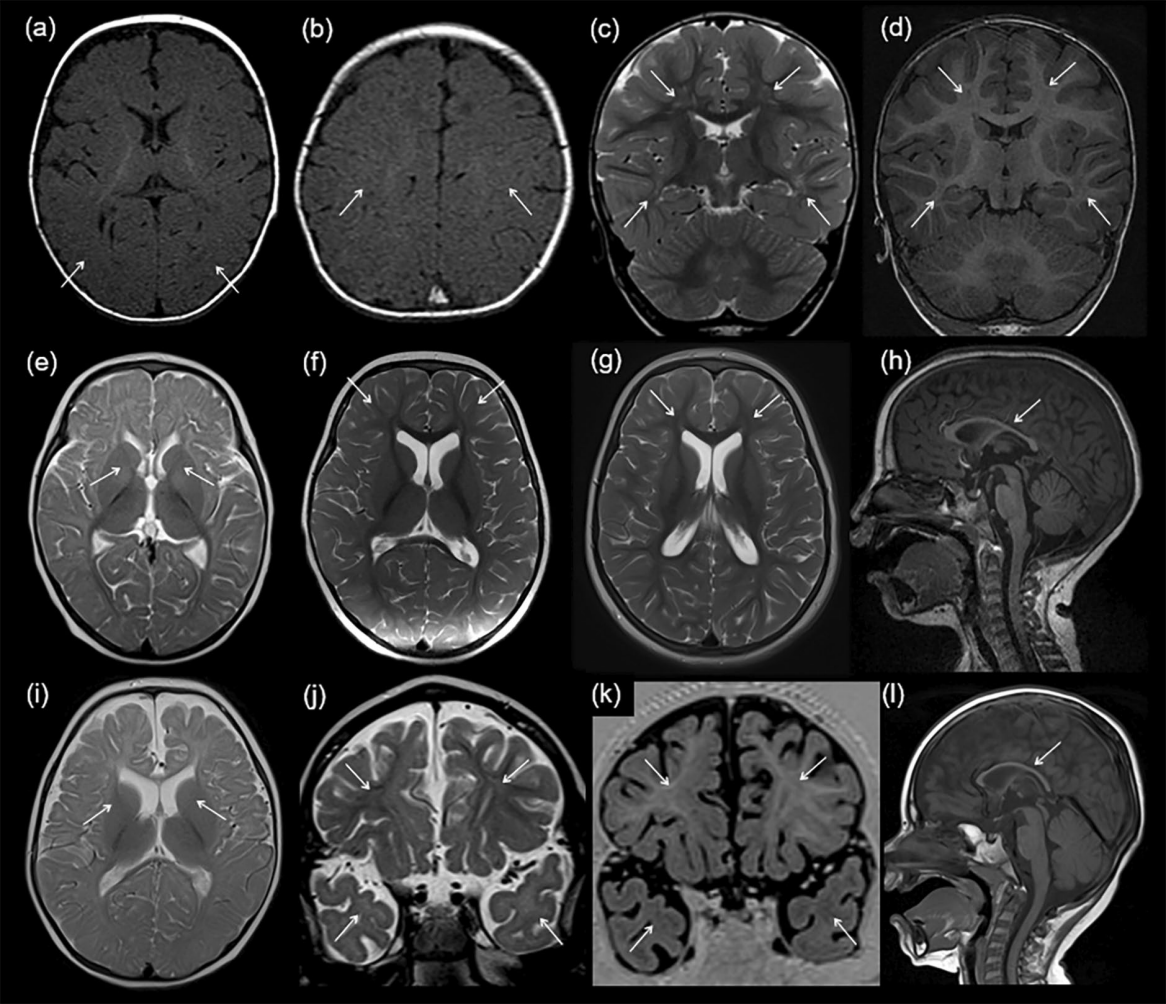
Mild hypomyelination of the diseases (early infantile epileptic encephalopathy 44 [DEE44], neurodegeneration with brain iron accumulation 5 [NBIA5], and X‐linked intellectual disability‐1 [MRX1]) previously not associated with hypomyelination. Axial T1‐weighted images of a 5‐month‐old patient with DEE44 do not present myelination of deep subcortical occipital white matter (a, arrows) or mid‐frontal white matter (b, arrows) as expected at this age. Follow‐up MRI of this patient with DEE44 at the age of 4 years shows slightly patchy frontal and temporal hypomyelination on coronal T2‐weighted (c, arrows) and T1‐weighted (d, arrows) images. Axial T2‐weighted image of a patient with NBIA5 at the age of 10 months does not show myelination of the anterior limbs of the internal capsule (e, arrows). At 22 months of age, maturation has progressed but there is still slight frontal hypomyelination on axial T2‐weighted image (f, arrows). At 3 years 8 months of age this T2‐weighted image of this patient with NBIA5 (g and h) shows patchy signal intensity in the anterior frontal white matter suggesting mild hypomyelination (g, arrows), and the sagittal T1‐weighted image demonstrates thinning of the corpus callosum (h, arrow). A patient with MRX1 at the age of 10 months lacks myelination of the anterior limbs of the internal capsule (i, arrows). At 2 years 9 months of age this patient with MRX1 presents hypomyelination of frontal and temporal white matter on the coronal T2‐weighted image (j, arrows) and T1‐weighted inversion recovery coronal image (k, arrows), and thinning of the corpus callosum on the sagittal T1‐weighted image (l, arrow).
